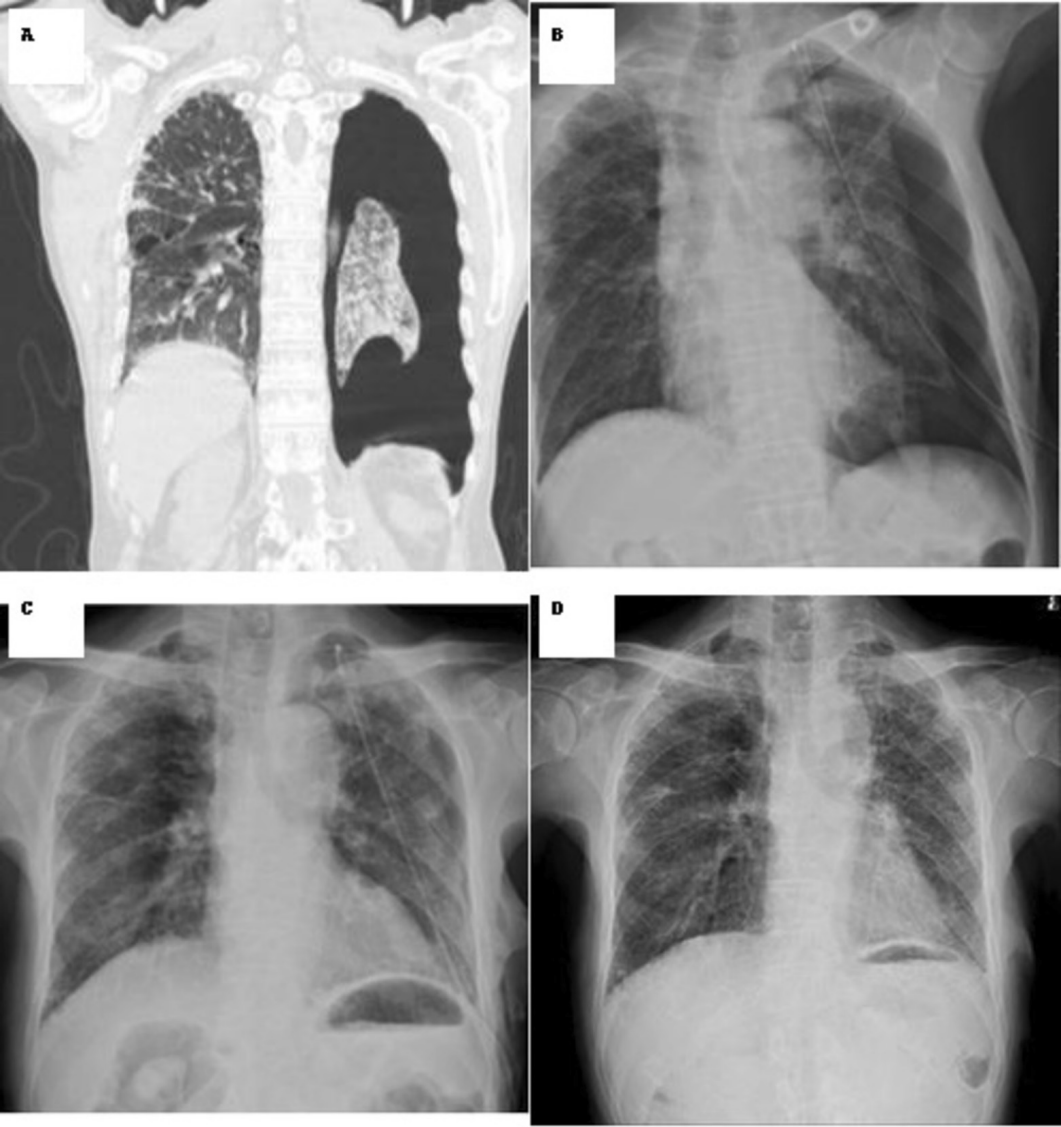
Representative case and radiologic course (panels A–D). Air‐leak cessation was confirmed by ≥ 24 h absence of bubbling on water seal at rest and with provoked cough, with stable chest radiography prior to tube removal. Pulmonary function tests were not performed owing to pneumothorax.
